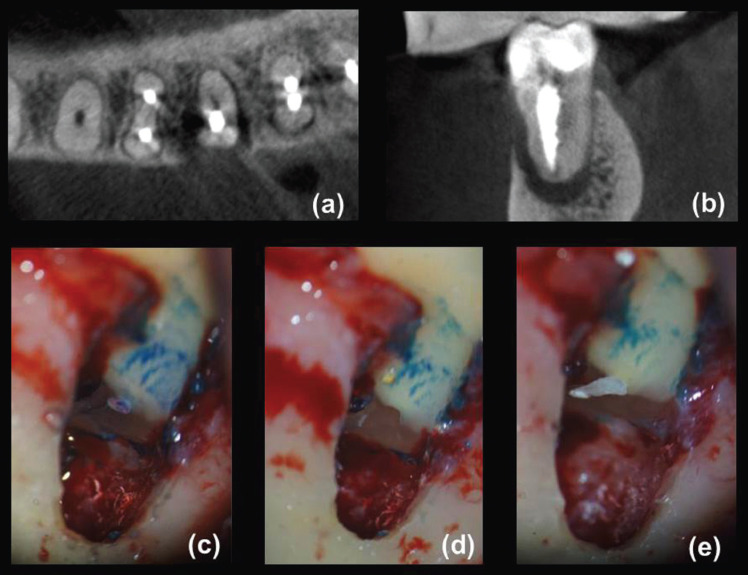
Mandibular left first molar endodontically treated with apical periodontitis accompanied by periodontal breakdown indicative of VRF. (a) Several fracture lines (solid arrows) and an accompanying periradicular bone defect with disruption of the buccal cortical plate can be observed on the axial CBCT scan. (b) Coronal CBCT scan showing buccal alveolar bone loss and J-shaped radiolucency (bone loss halo). (c) The surgical procedure revealed the presence of unprepared lateral ramifications of the root canal system in the apical third of the distal root (dashed arrows). (d) After root-end cavity preparation, no fracture lines were observed on resected root surface. (e) Root-end filling of the exposed canal was performed using EndoSequence root repair material.
